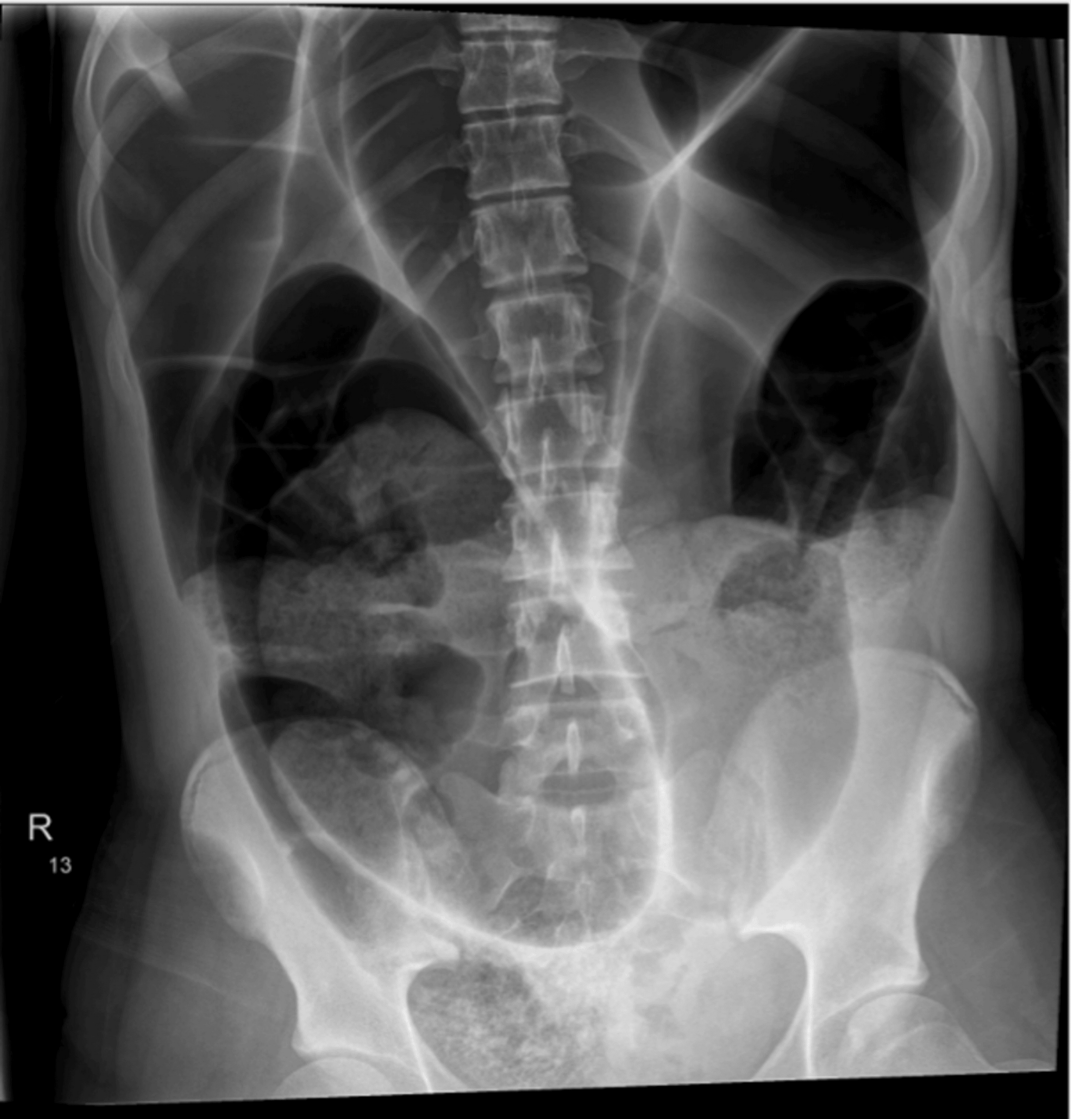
An abdominal radiograph showing a coffee bean sign
